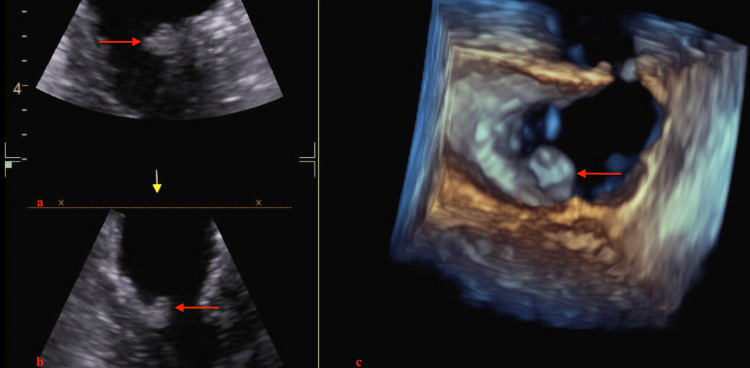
TEE (a, b) Mid-esophageal two-chamber views revealing mitral valve vegetation (indicated by red arrows). (c) Reconstructed 3D view showing mitral valve vegetation (red arrow). TEE, transesophageal echocardiography
